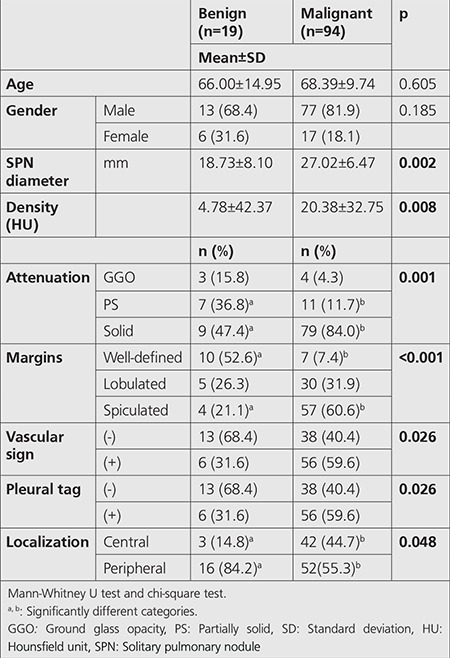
Demographic findings of patients and computed tomography findings of benign-malignant solitary pulmonary nodules
